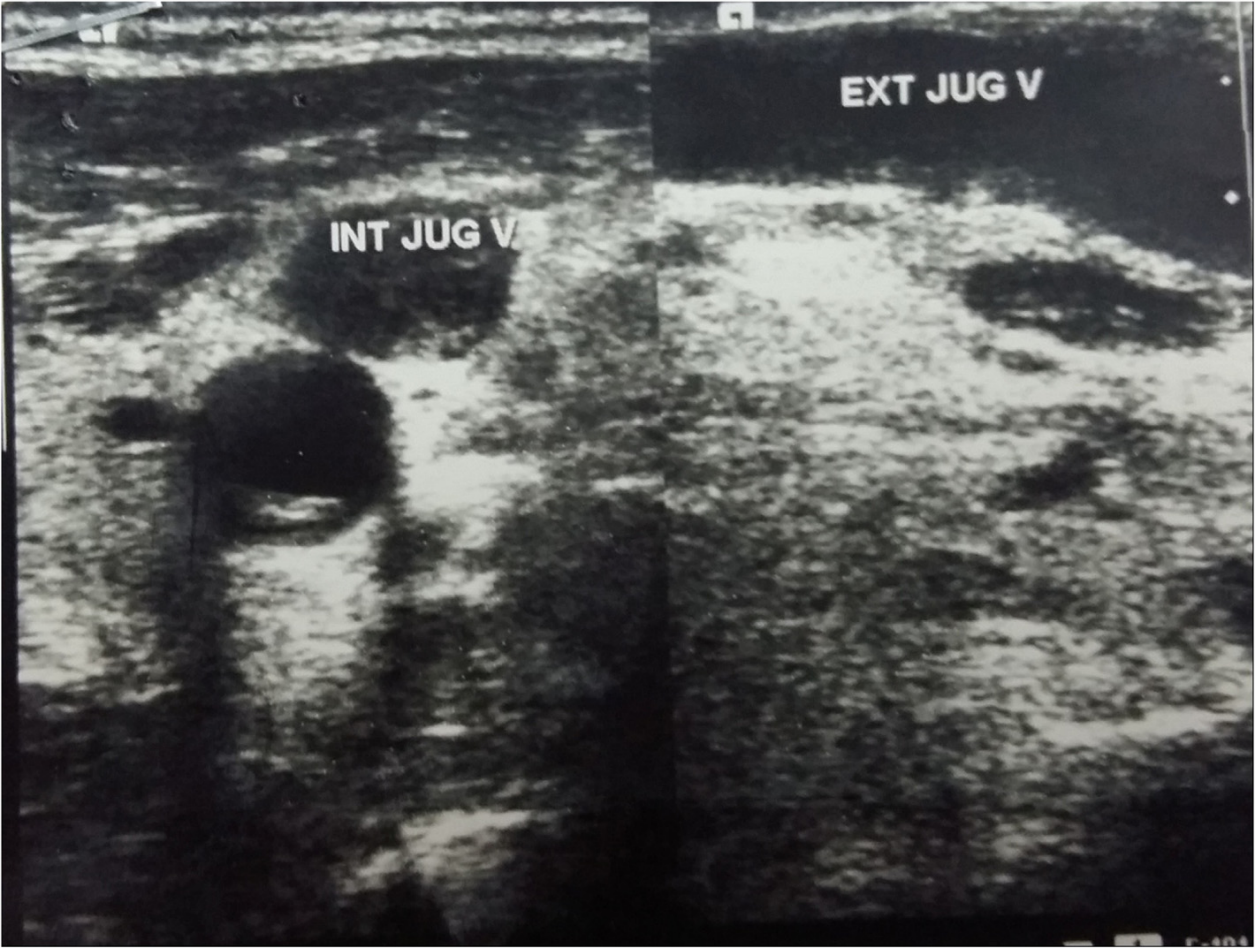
Ultrasound duplex scan of neck showing thrombosis of internal jugular vein. *EXT JUG V* external jugular vein, *INT JUG V* internal jugular vein
